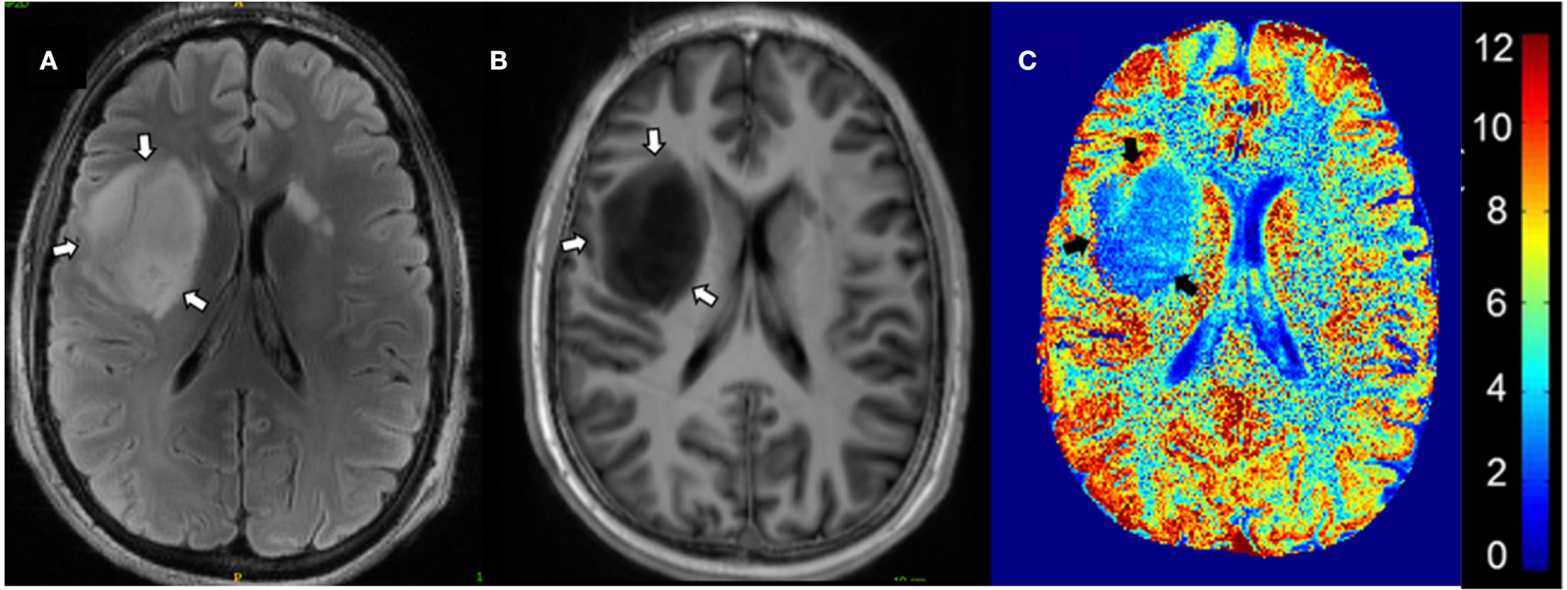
Glutamate-CEST imaging of a diffuse astrocytoma. (A) 3T FLAIR with tumors identified by white arrows. (B) 7T FLAIR with tumors identified by white arrows. (C) Glutamate-CEST contrast map with tumors identified by black arrows and CEST contrast gradient on the right. Figure used with permission from Neal et al., 2019 (60).
